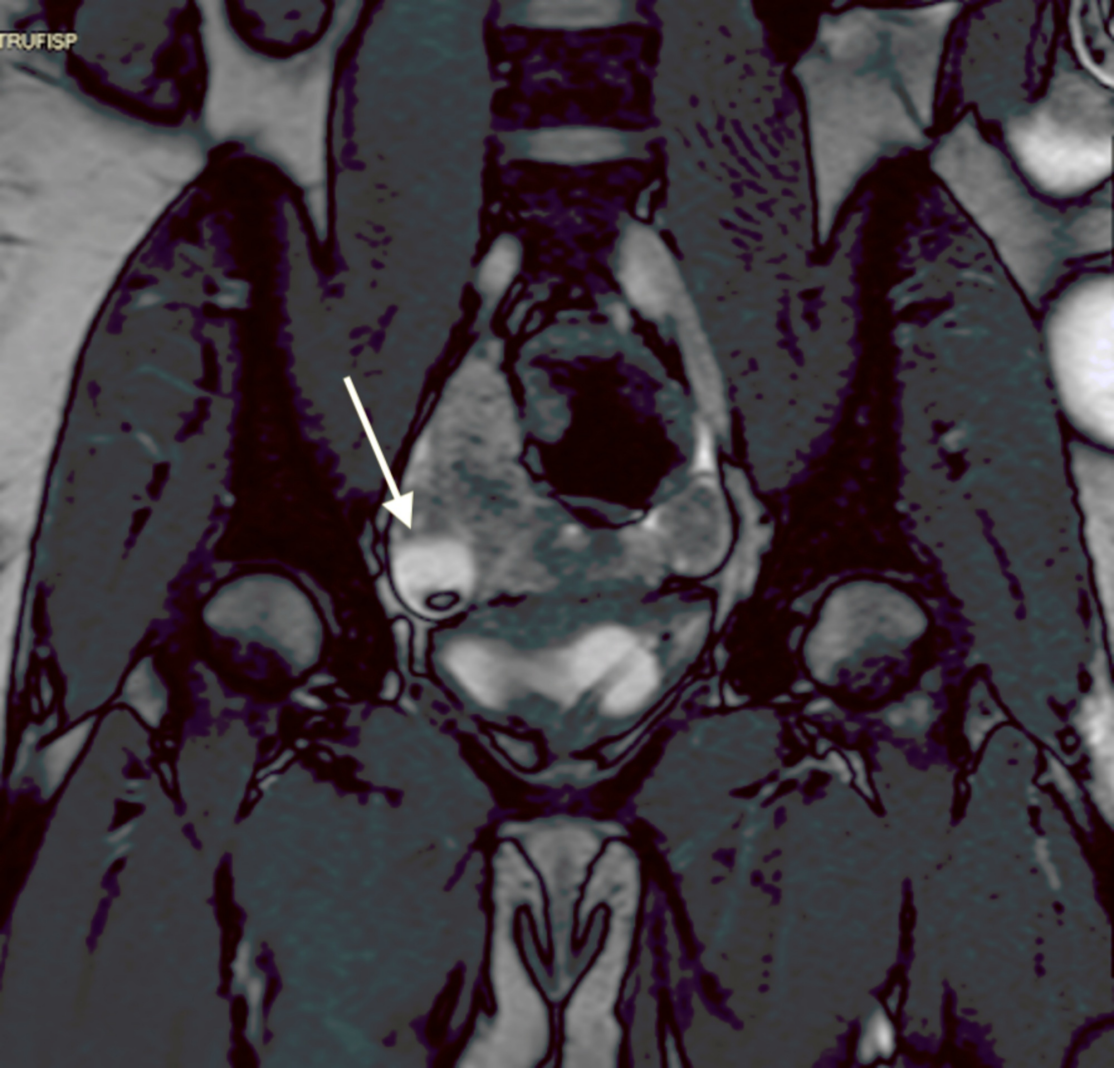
Coronal MRI Pelvis with IV Contrast
